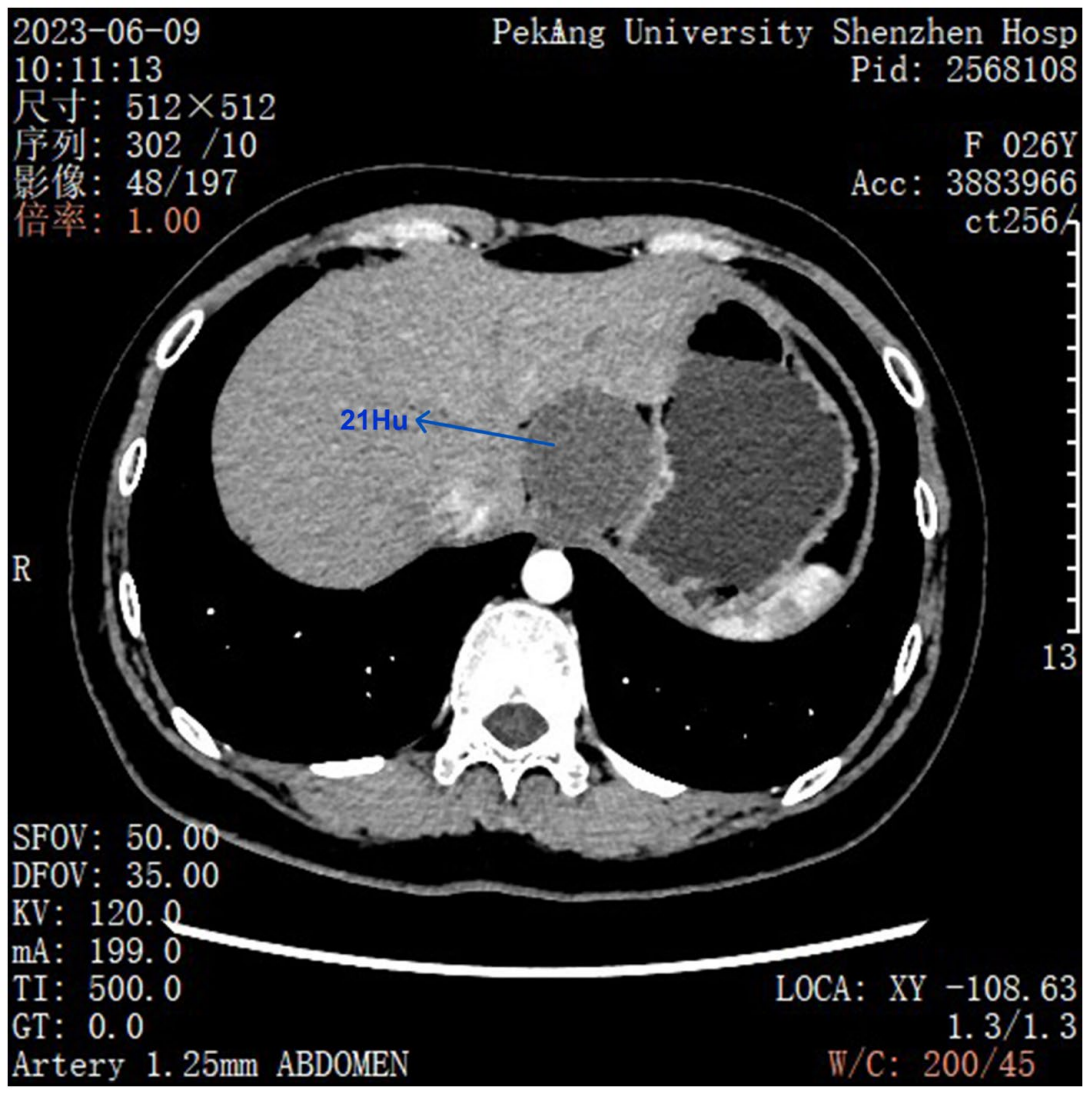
Preoperative CT scan revealed a quasi-circular low-density shadow in the hepatogastric space, approximately 48 mm*46 mm in size, with a CT value of approximately 21 HU. Slight calcification was visible at its edge. No significant enhancement was observed after contrast administration, while the boundary remained clear. Compression changes affected the adjacent left lobe of the liver and the gastric wall.
